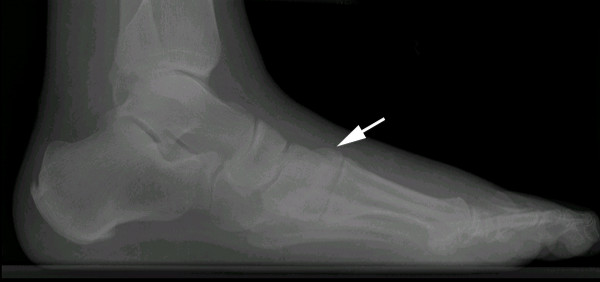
Lateral weight-bearing film of the right foot. This radiograph shows the characteristic "step-off" point (arrow) caused by dorsal displacement of the 2^nd ^metatarsal relative to the medial cuneiform.
